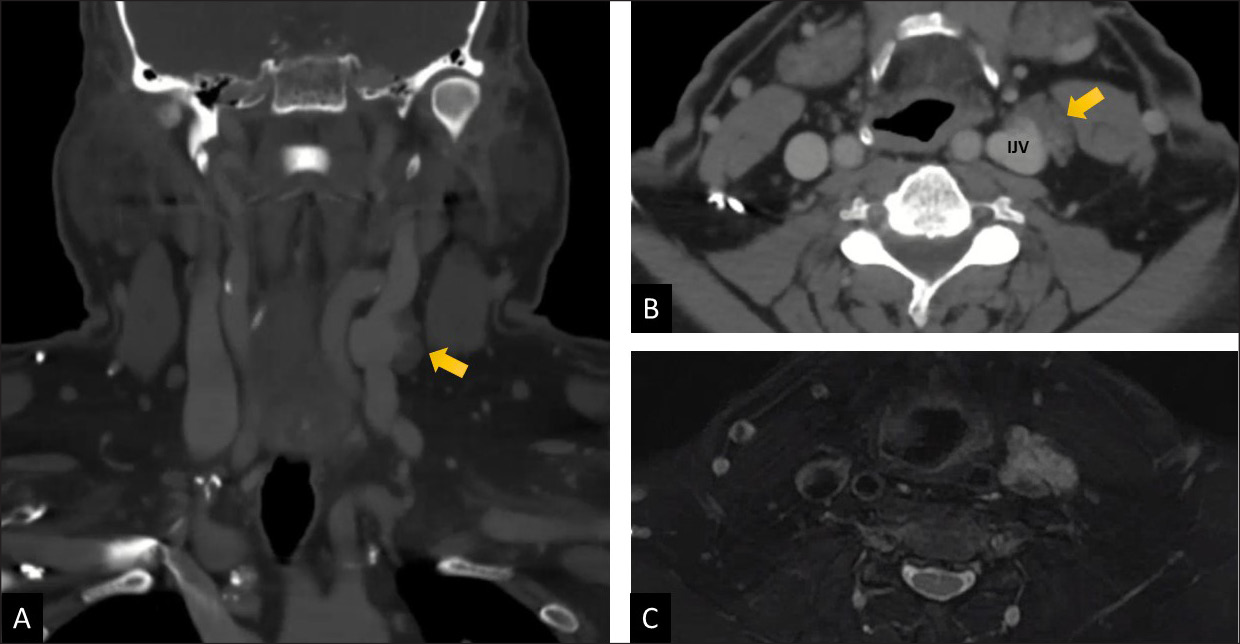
Preoperative imaging of internal jugular venous malformation. (A) Coronal view of computerized tomographic scan. (B) Axial view of computerized tomographic scan. (C) Magnetic resonance imaging showing hyperintensity of lesion in T2.
